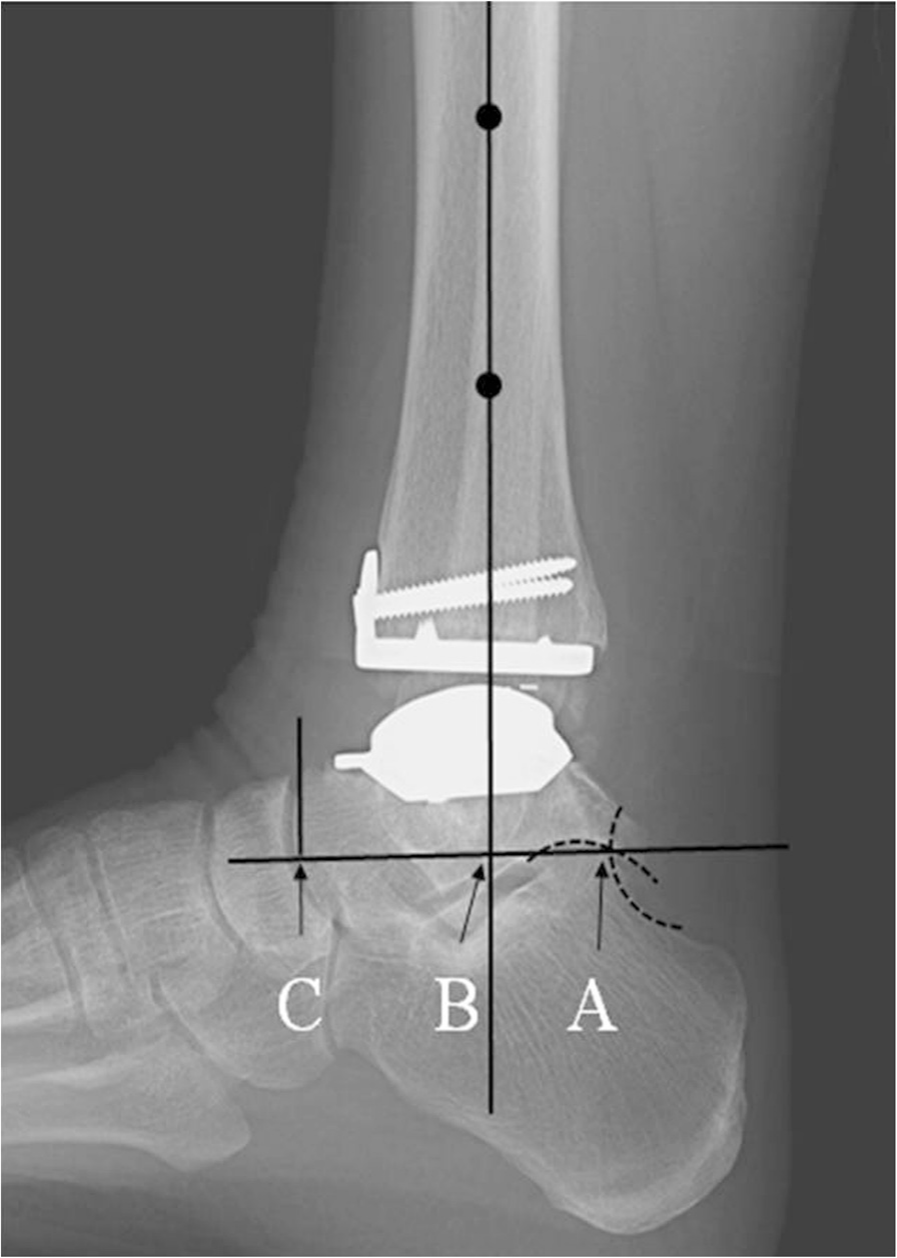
Lateral radiograph showing the measurements used to determine the postoperative tibiotalar ratio (tibiotalar ratio = AB / AC x 100). The posterior talar point (point A) was defined as the intersection between the contours of the posterior subtalar articular surface and the posterosuperior calcaneal cortex, and the talar reference line was defined as the line through point A parallel to the floor. The anterior talar point (point C) was defined as the vertical projection of the most anterior aspect of the talus onto the talar reference line. Longitudinal talar length was defined as the length AC, and the distal tibial axis was defined as the longitudinal middisecting line of the distal tibial shaft determined at 5 and 10 cm above the ankle.
